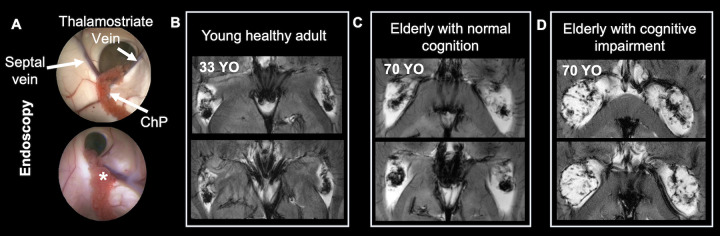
Ultra-high field (7T) MRI provide details of the ChP sub-structures. (A) Invasive endoscopy images showed ChP glomus with granular appearance tangling with supplying arteries/drainage veins. It also revealed cyst (asterisk) structure of the ChP (Adapted from Ref [153]). ChP images using 2D GRE on 7T MRI of (B) a young healthy adult, (C) an old subject with normal cognition, and (D) an old subject with cognitive impairment, respectively.
